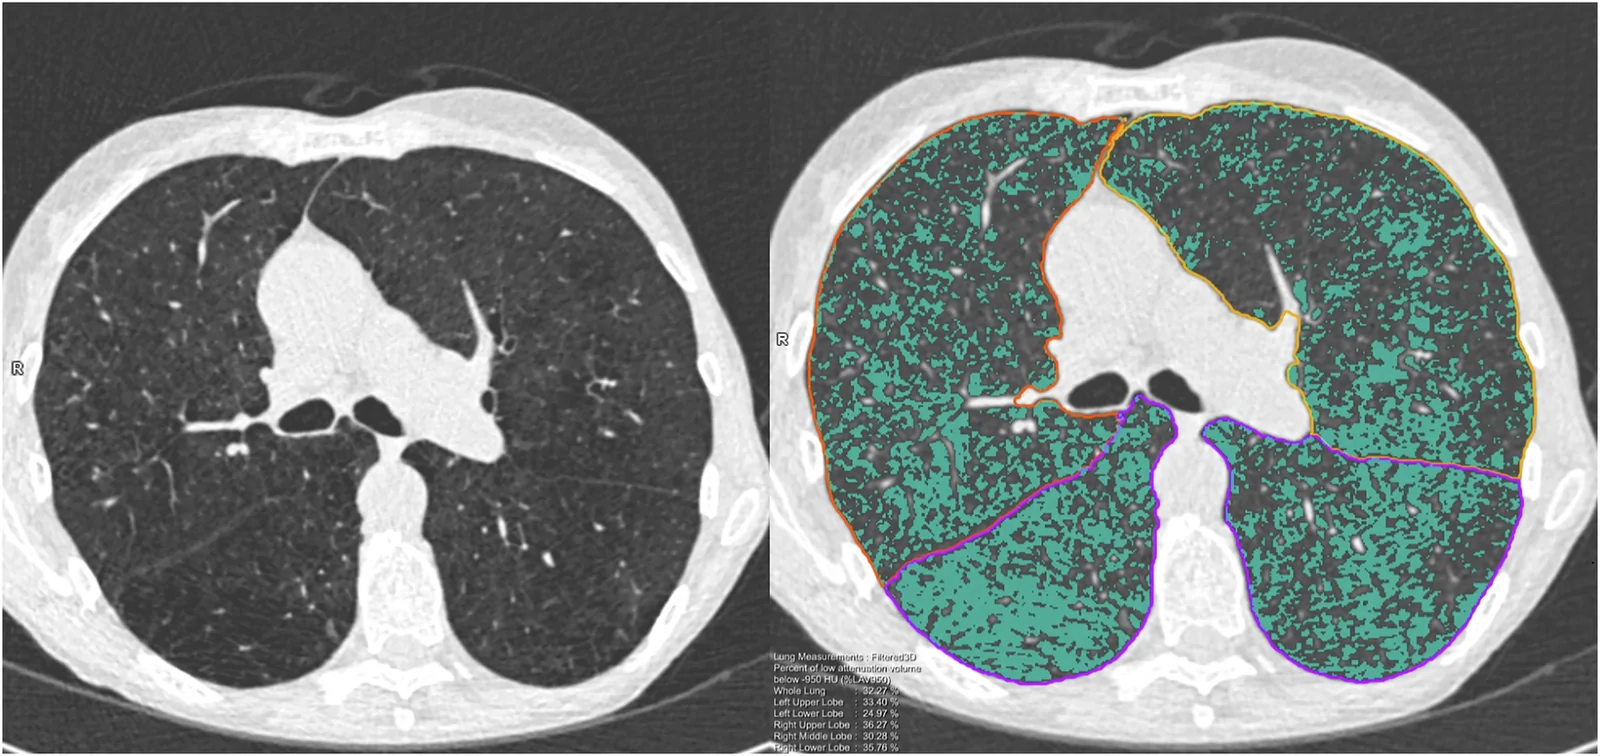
Example of subject with a high amount of emphysema. Left: CT slice. Right: emphysema segmentation, displayed in cyan. Colored outlines indicate lung lobes
